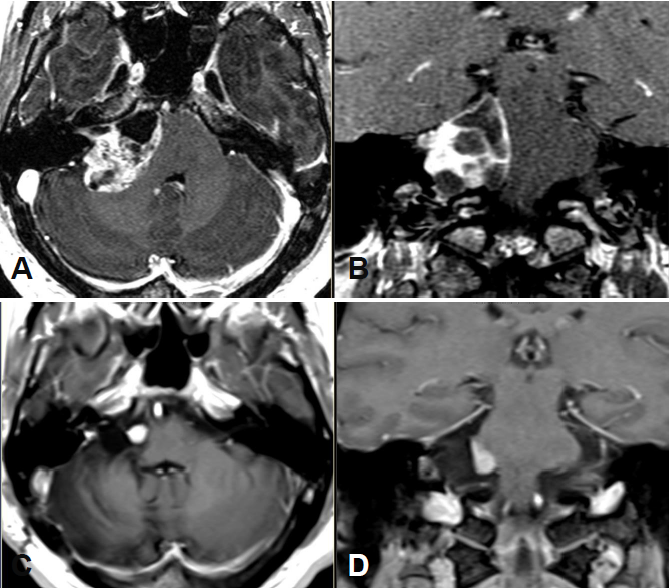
Preoperative MRI, T1-w + CM; A transversal; B coronal. MRI 9 years after surgery, T1-w + CM; C transversal; D coronal. Intensive homogeneous contrast enhancement of the residual tumor.
